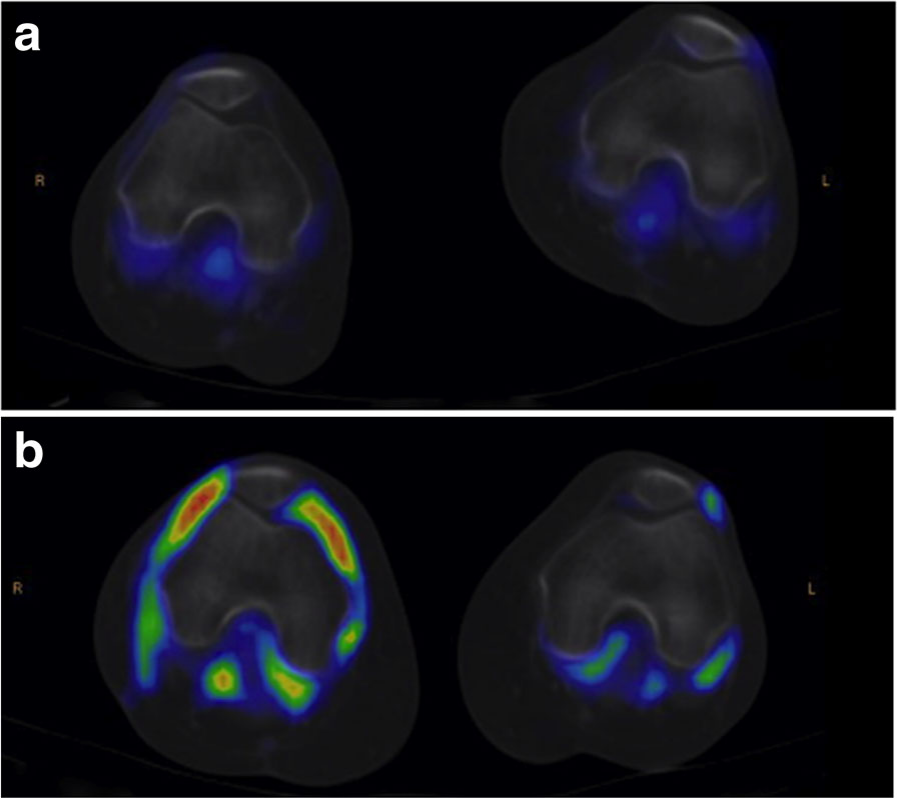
Progression of disease in a 66 year-old man. The top row (a, fused transaxial PET/CT images) show no abnormal uptake in the knees, whereas the PET/CT performed at week 16 show bilateral synovitis, especially in the right knee (b)
